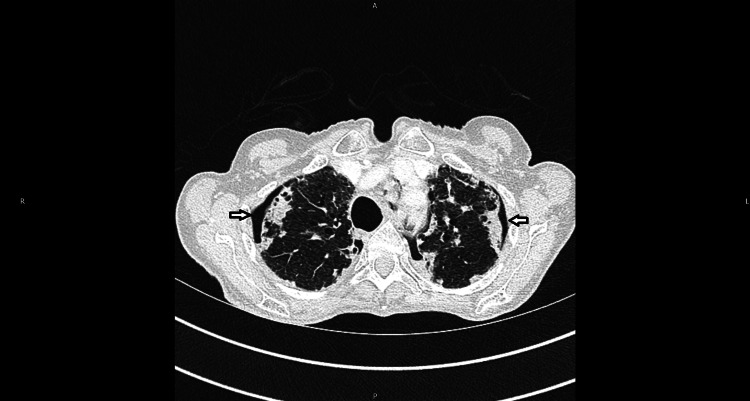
CT thorax demonstrating biapical pleural thickening, subpleural fibrosis and traction bronchiectasis. Note the bilateral pneumothoraces (arrow).
